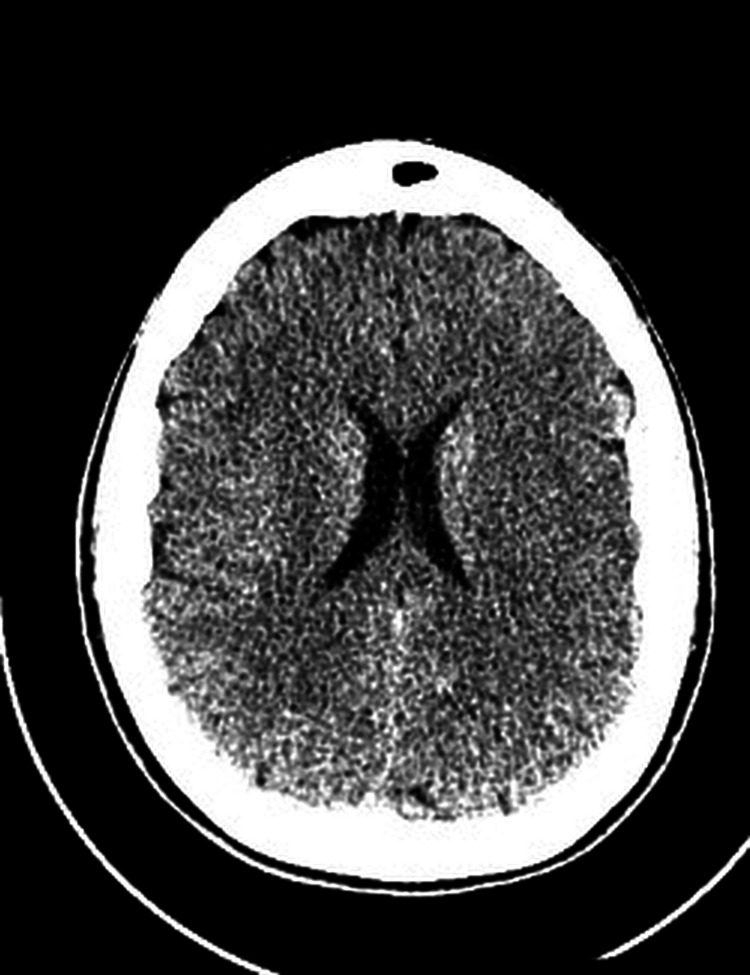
Noncontrast CT of the brain (axial view). The image obtained at the level of the lateral ventricles demonstrates no acute intracranial hemorrhage, mass effect, midline shift, or findings suggestive of HIV-associated opportunistic central nervous system infection. The ventricular system is within normal limits for the patient’s age.
